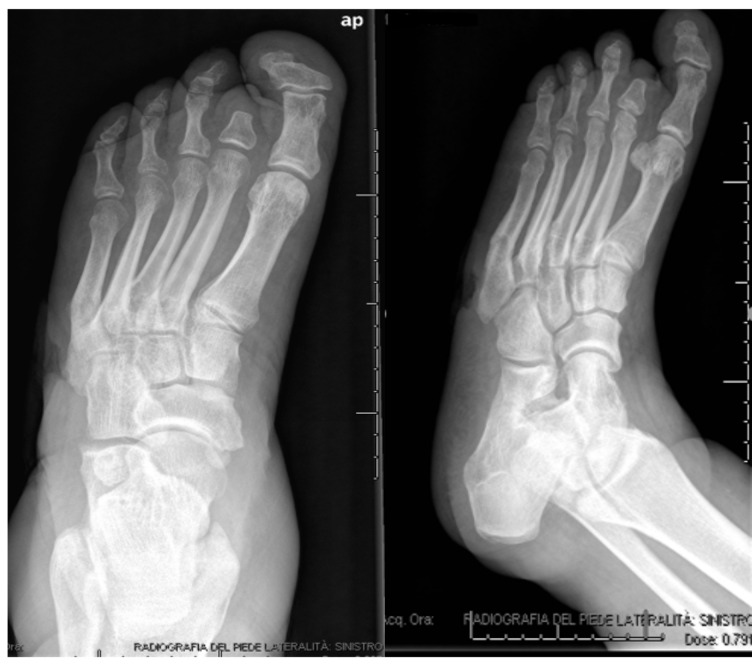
Pre-operative X-rays showing the affected cuboid.
